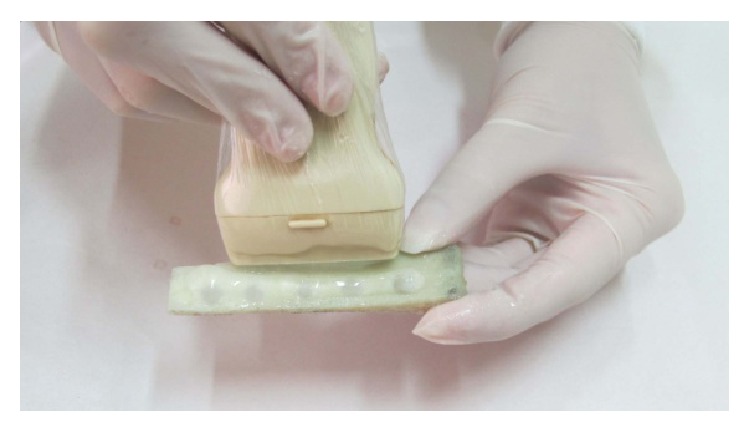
Ultrasonic gel was applied on the intact surface on the linear ultrasonic probe that was placed on the block.
